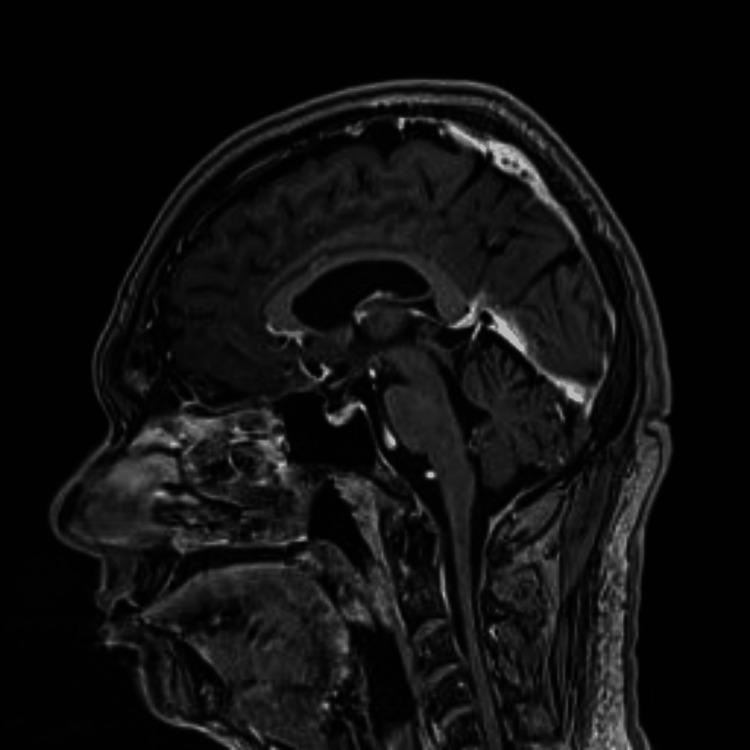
MRI T1-weighted midline sagittal cut
